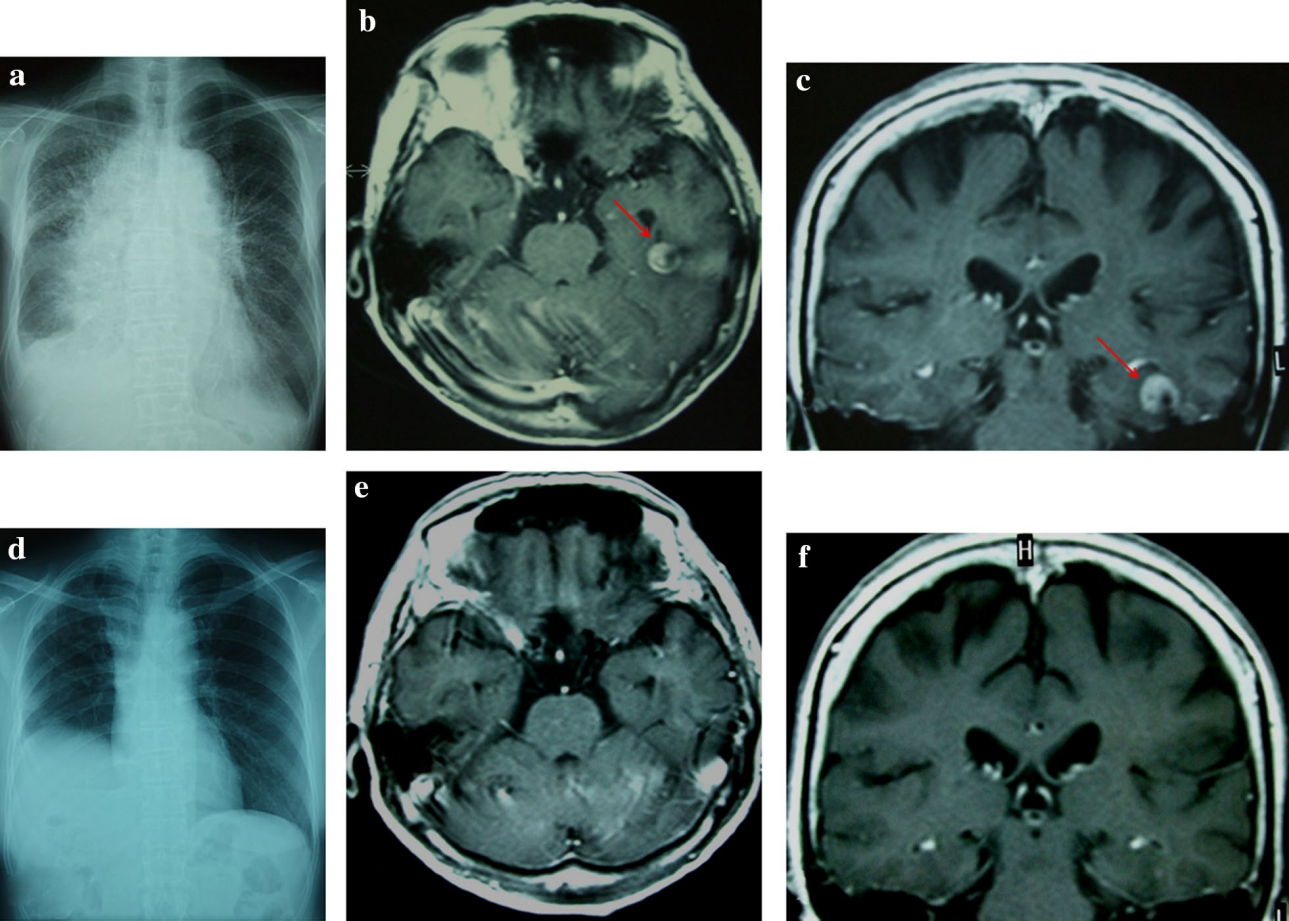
Chest X-ray examination and T1-weighted magnetic resonance imaging (MRI) with gadolinium. a
